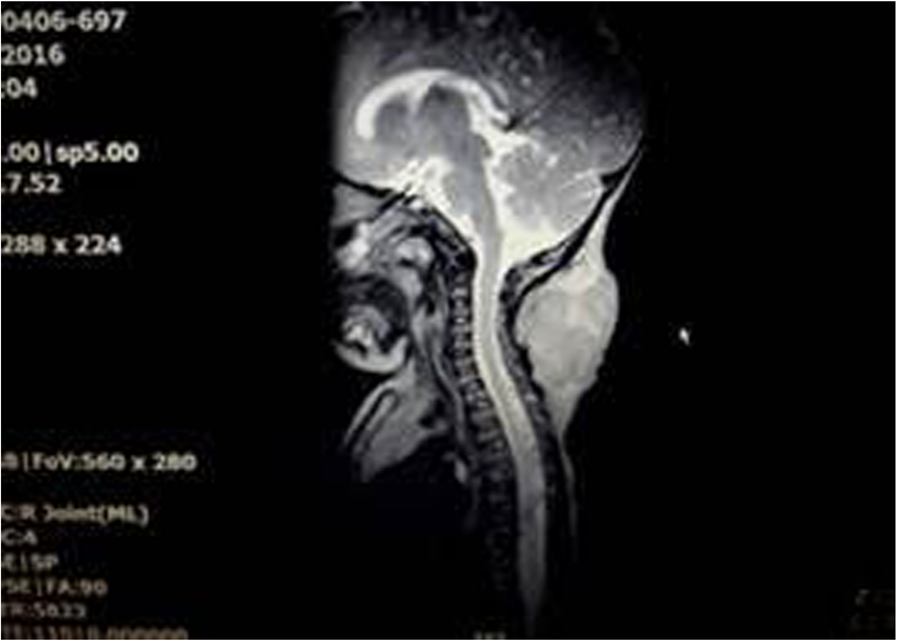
Magnetic resonance imaging of soft tissue mass in the neck – the contrast enhancement on T2-weighted sequence
